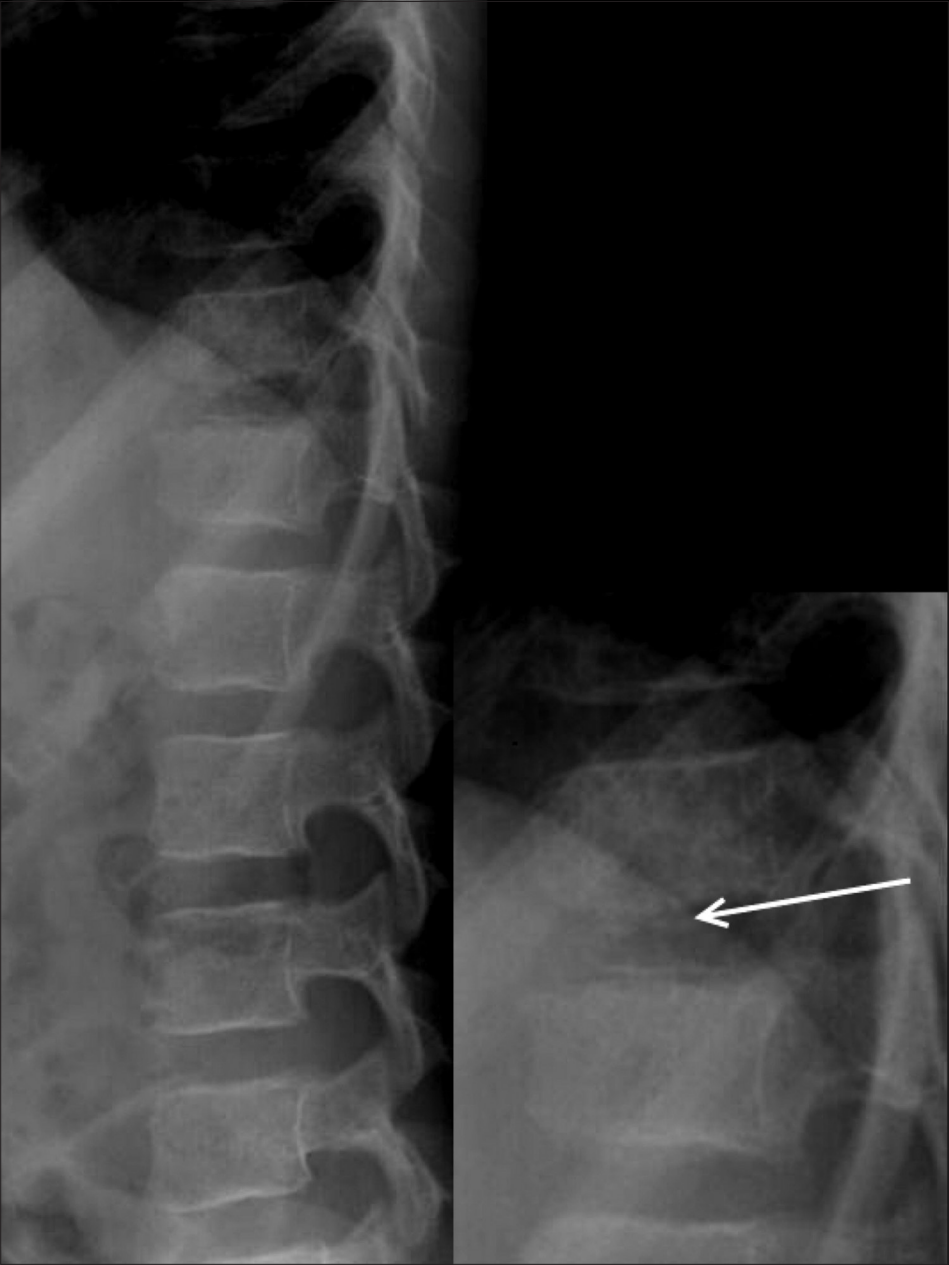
Lateral dorsolumbar spine radiograph obtained 2 weeks after presentation shows partial resolution of the calcification (arrow)
